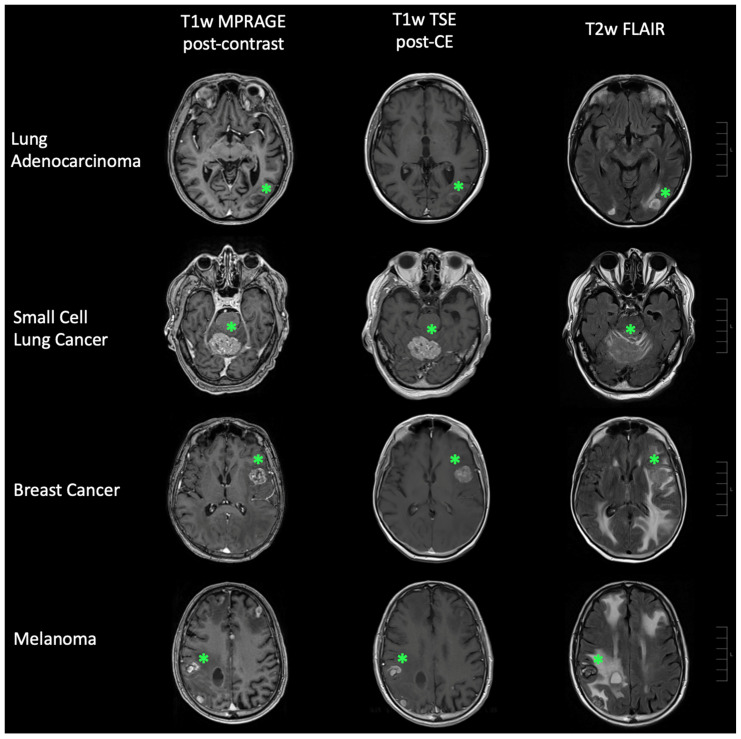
Representative cases of brain metastases. Brain metastases (green star) linked to lung adenocarcinoma, small cell lung cancer, breast cancer, and malignant melanoma are depicted. The left panel shows T1-weighted (T1w) MPRAGE post-contrast-enhancement (CE) imaging, the middle panel displays T1w TSE post-CE imaging, and the right panel features T2-weighted (T2w) FLAIR imaging. Scale bars (right side of each row) correspond to 10 mm per unit.
